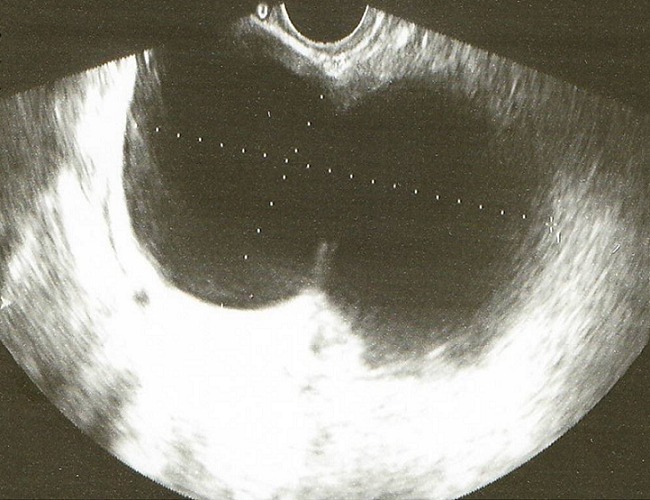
The sonographic evaluation showed a 100/52mm right oblong adnexal cyst suggesting a hydrosalpinx
